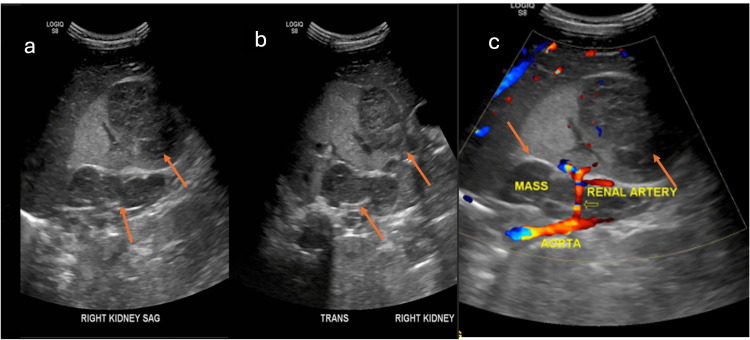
Sagittal and transverse (a,b) of the right kidney shows a well-circumscribed heterogeneous predominantly hypoechoic lesion in the lower pole of the right kidney with another hypoechogenic lesion projecting in the retroperitoneal region along the medial aspect of the right kidney. (c) Doppler image shows no definite vascularity within the lesion (orange arrows).
